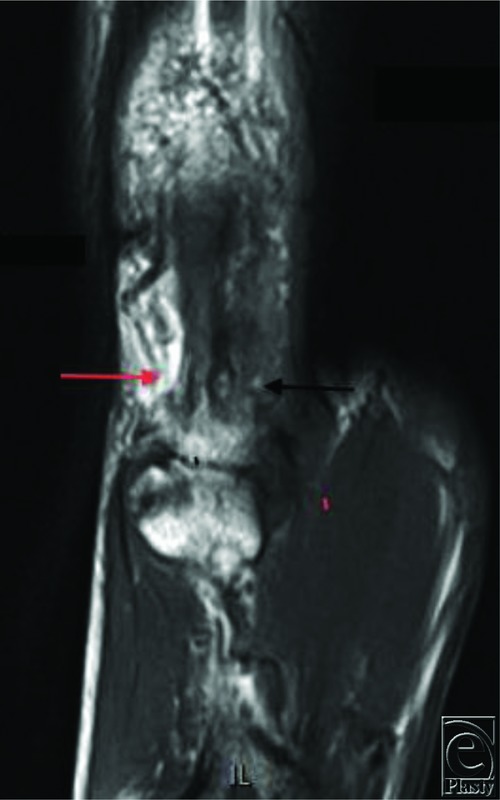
Magnetic resonance imaging of the left thumb. STIR images showing hypointensity of the ulnar digital vessel (black arrow). Note the hyperintense signal of the patent radial digital artery (red arrow). This finding was not considered diagnostic of arterial occlusion.
